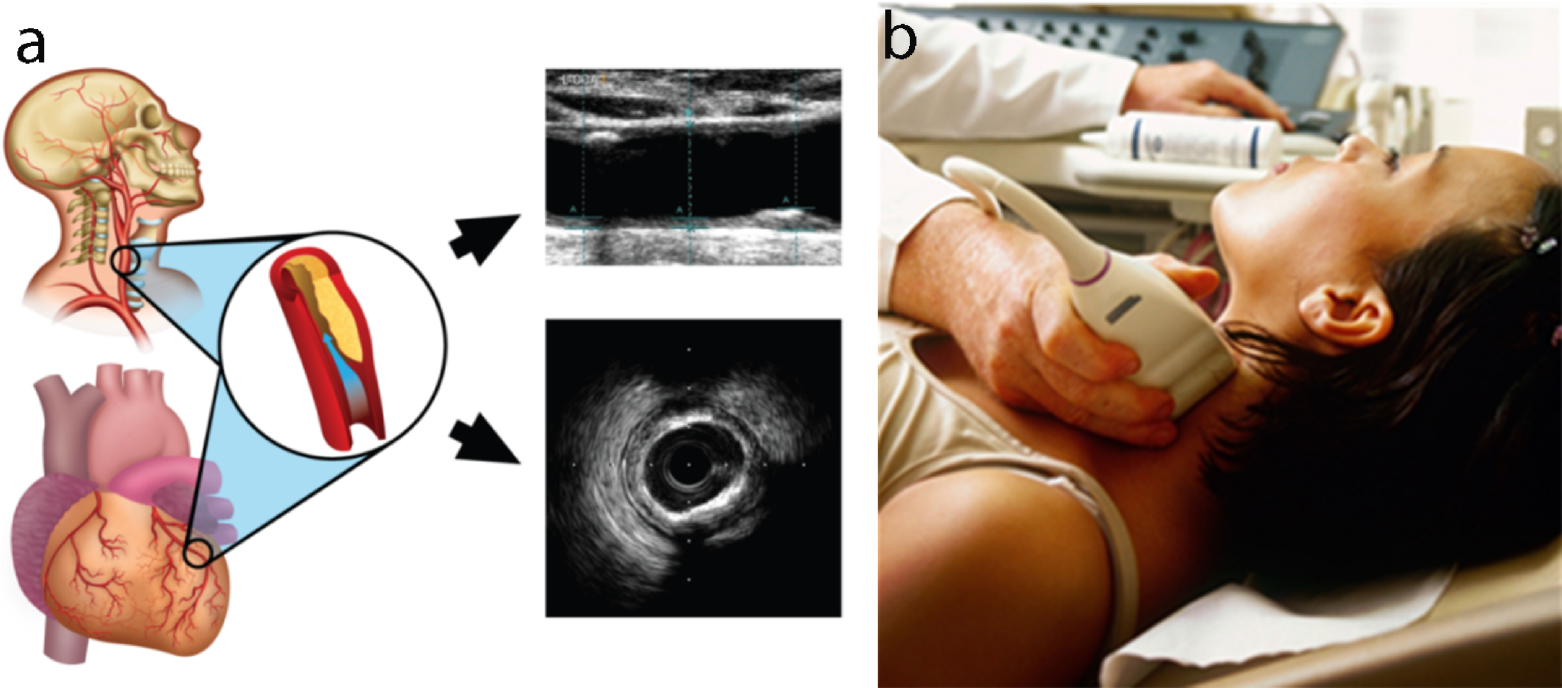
The carotid artery examination using intravascular ultrasound
